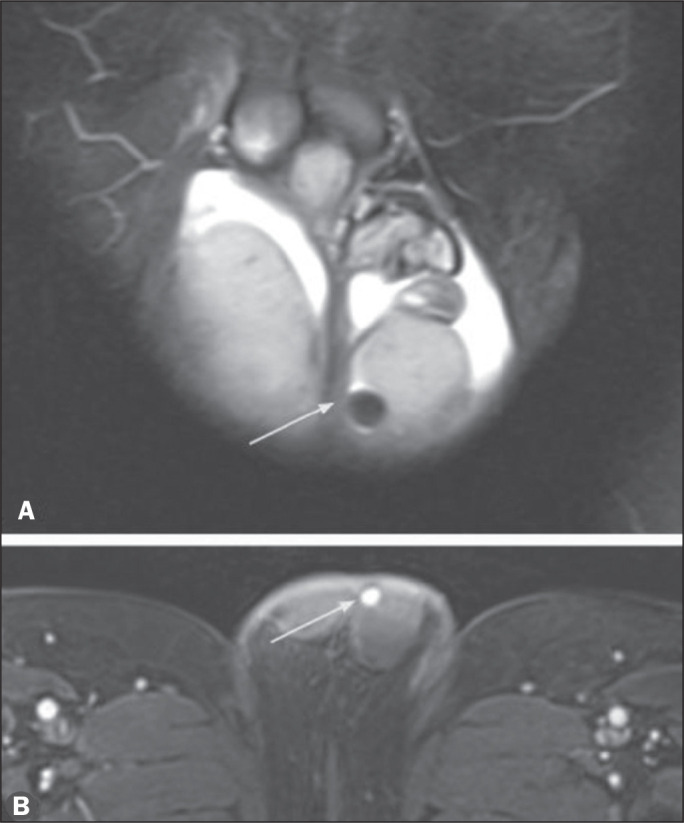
Left-sided adenomatoid tumor. Coronal T2-weighted image with fat
